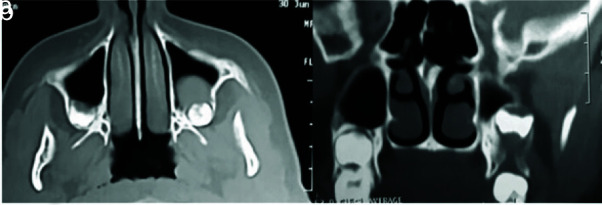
a: The axial view of the CT scan showing the position of the dental germ of the third molar and its relation to the maxillary sinus and the thin posterior cortical bone
